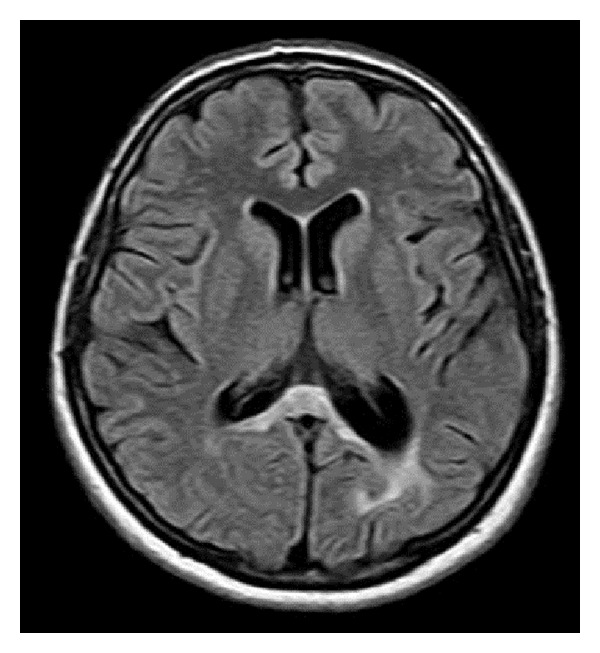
FLAIR-weighted cranial MRI demonstrating important reduction in the lesion following treatment.
